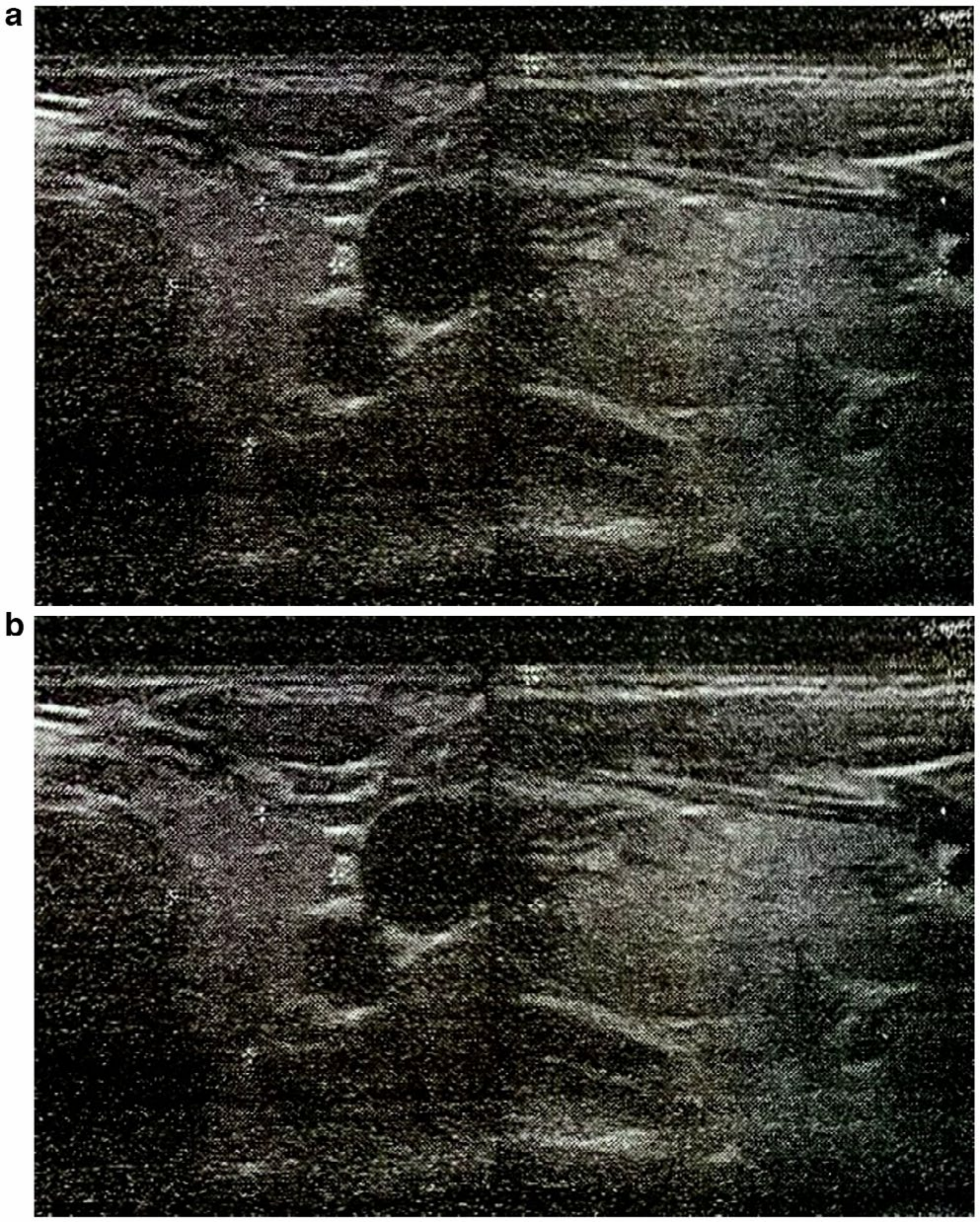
(a and b) Ultrasound showing bilateral lymphadenopathy.
